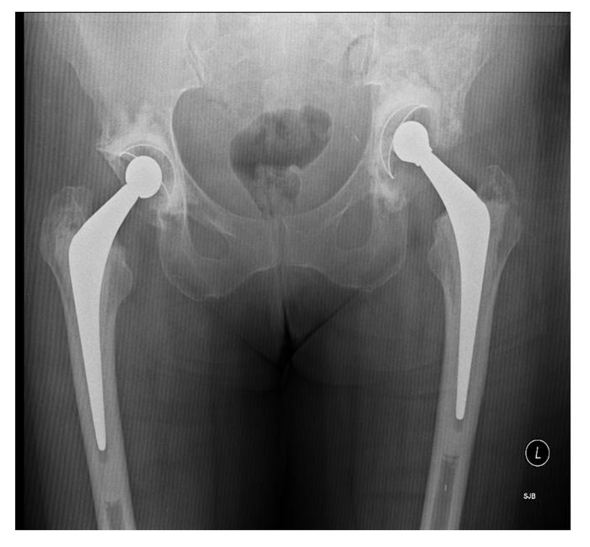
Eight month follow-up X-ray showing isolated periacetabular bone loss in left hip.
